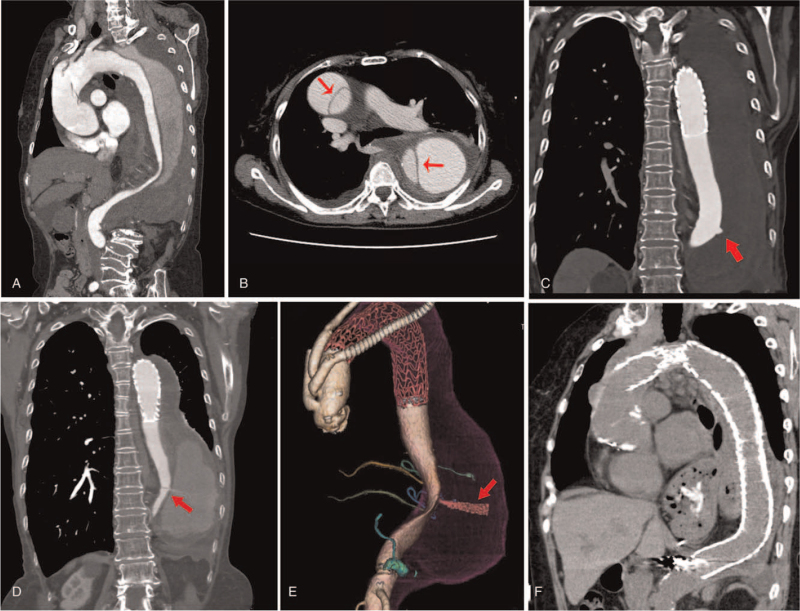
Contrast-enhanced CT performed on admission. A. Acute type A dissection of the ascending aorta and type B aortic dissection surrounded by a hematoma. B. Axial view showed the occurrence of TAAD and TBAD at the same time (arrow). C. Coronal view showed the thrombosed false lumen and a new aortic ulcer-like change (arrow) in 1-month follow-up. D. Coronal view showed the dilated false lumen and jet-like flow from the developing ulcer-like projection (arrow). E. Volume-rendered chest CT angiographic image showed jet-like flow (arrow) into the enlarged false lumen. F. Follow-up CT performed 1 year after the endovascular intervention and the image showed a correctly positioned endograft with a thrombotic and shrunken false lumen. CT = computed tomography, TAAD = type A aortic dissection, TBAD = type B aortic dissection.
